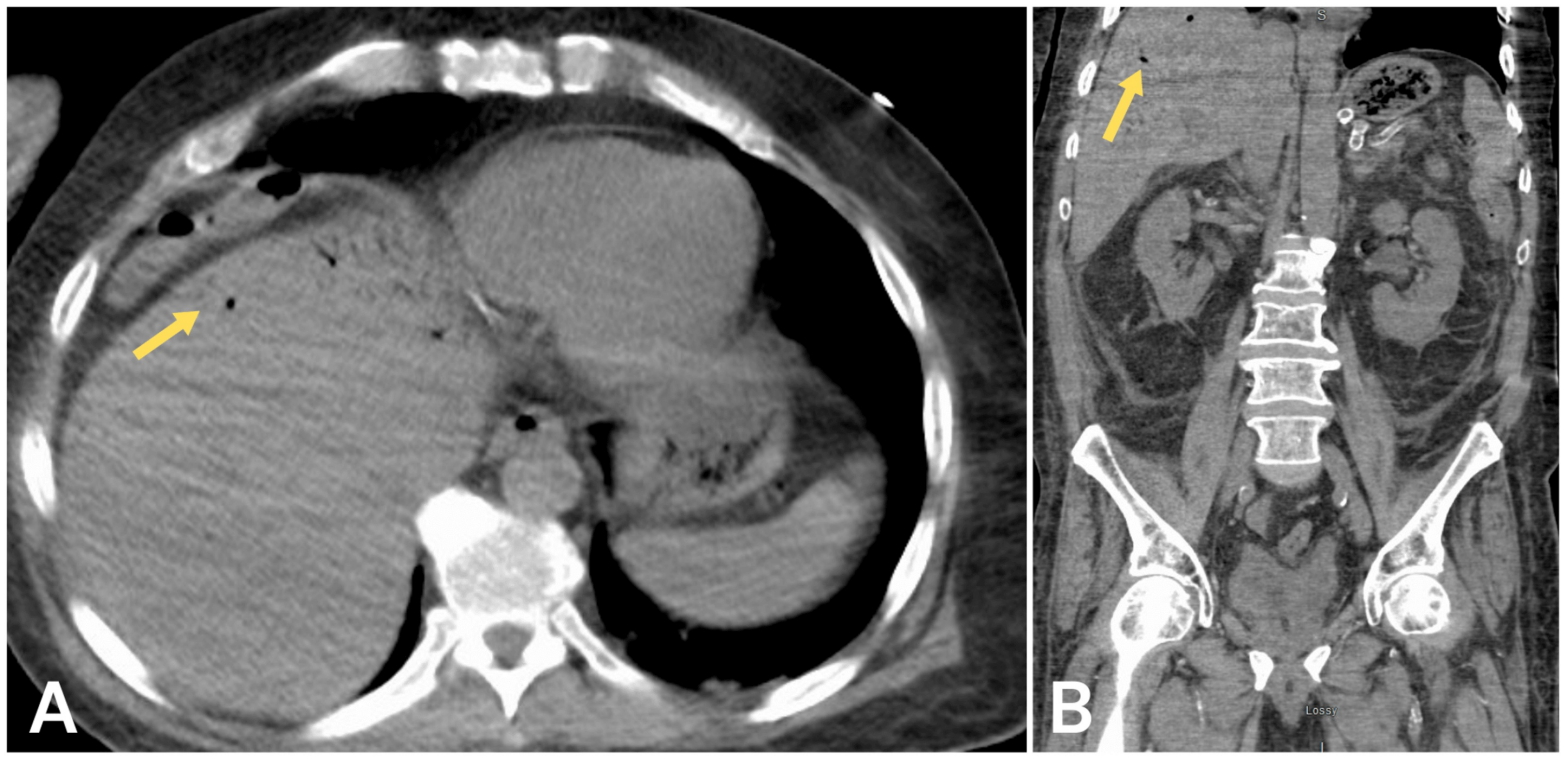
CT imaging revealing branching air/lucencies in the periphery of the liver parenchyma most consistent with portal venous air. Bowel ischemia is a common cause of portal venous air. (A) Axial view. (B) Coronal view.
